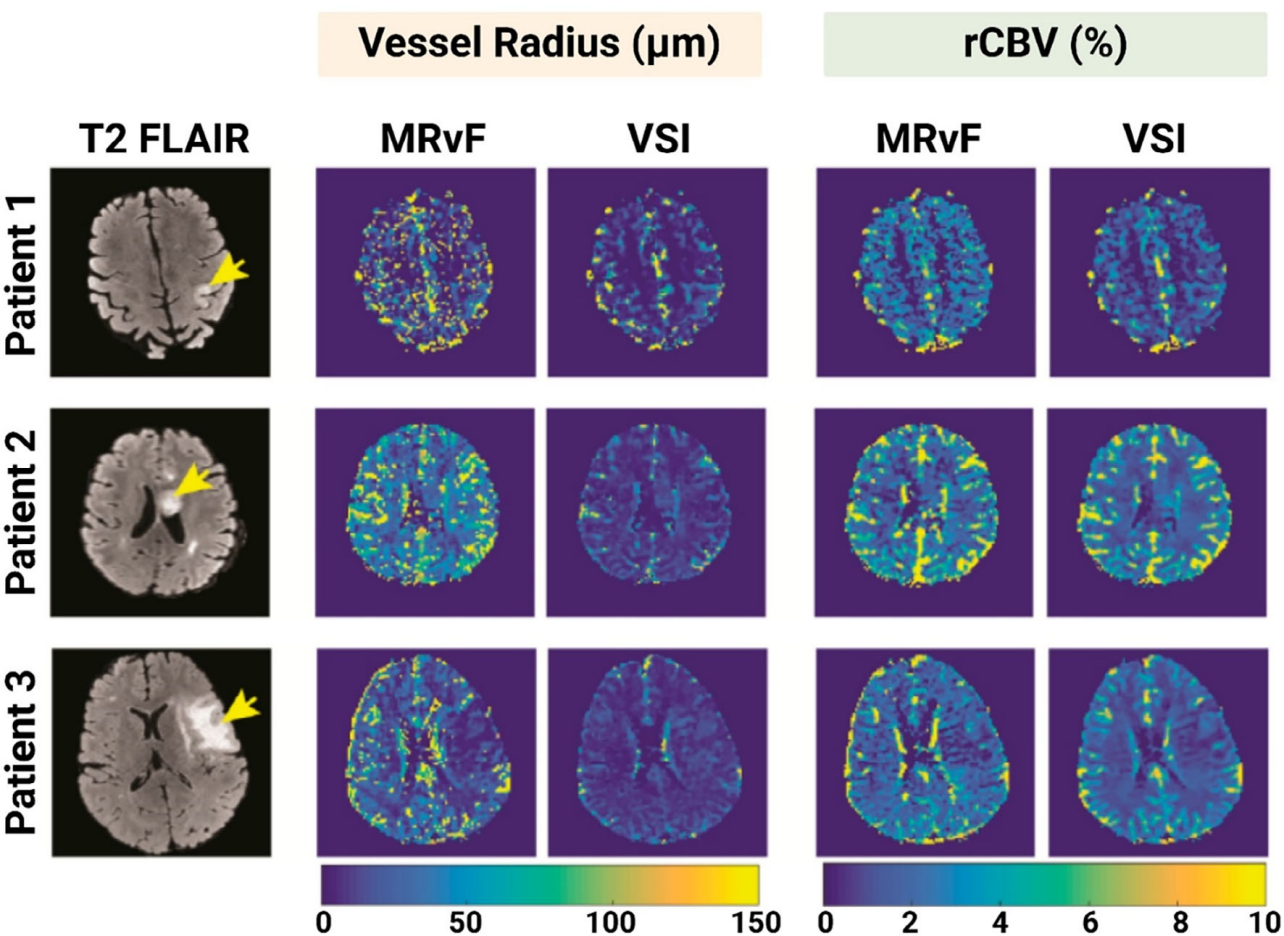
Comparison of R (μm) and rCBV (%) maps derived from MRvF and the Vessel Size Imaging (VSI) method for three patients with gliomas. T_2_ FLAIR images (left column) highlight tumor regions (yellow arrows). The MRvF‐derived maps (middle columns) show vessel radius and rCBV values alongside those derived from VSI (right columns). Adapted from Venugopal et al. [34], *Cancers*, 2023.
